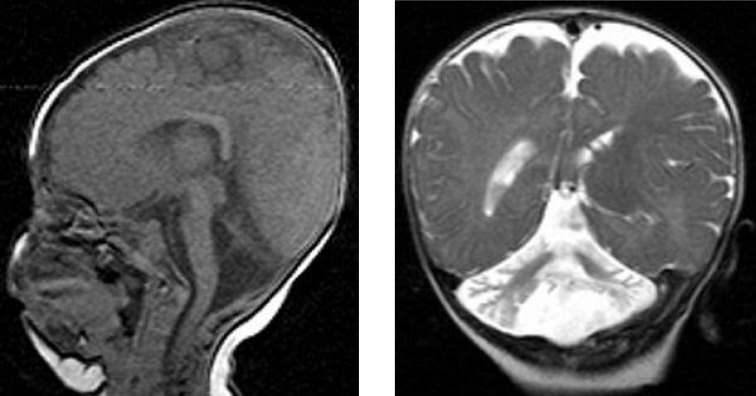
Coronal T2 (A) and sagittal T1 (B) magnetic resonance images showing cerebellar vermis hypoplasia and a generalized reduction in myelination
